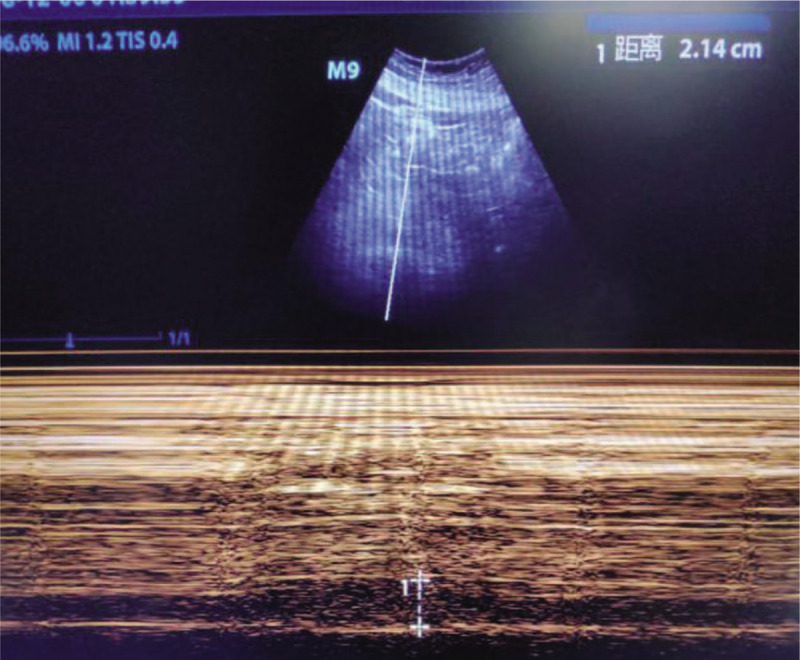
B-mode ultrasonography shows the end-inspiratory width of the inferior vena cava was 2.92 cm.
